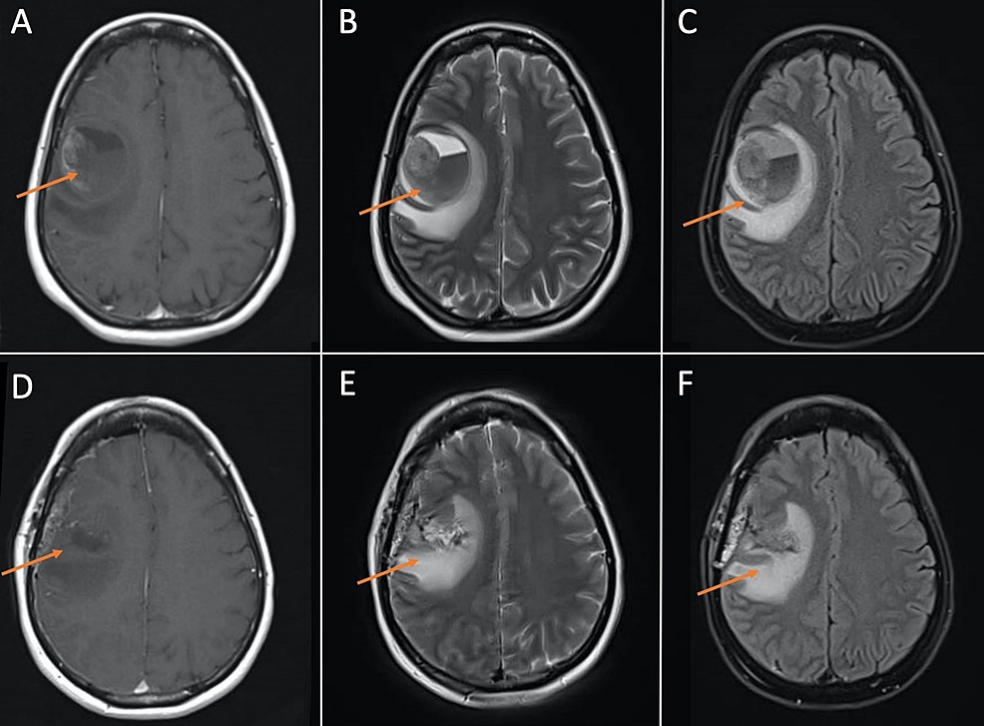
MRI brain demonstrating large hemorrhagic cavity in the right frontal lobe with an enhancing focus along the right superolateral margin representing a hemorrhagic mass. (A), (B), and (C) are pre-operative T1, T2, and FLAIR images, respectively. (D), (E), and (F) are post-operative T1, T2, and FLAIR images, respectively, demonstrating resection of mass and post-surgical changes. FLAIR: fluid-attenuated inversion recovery.
